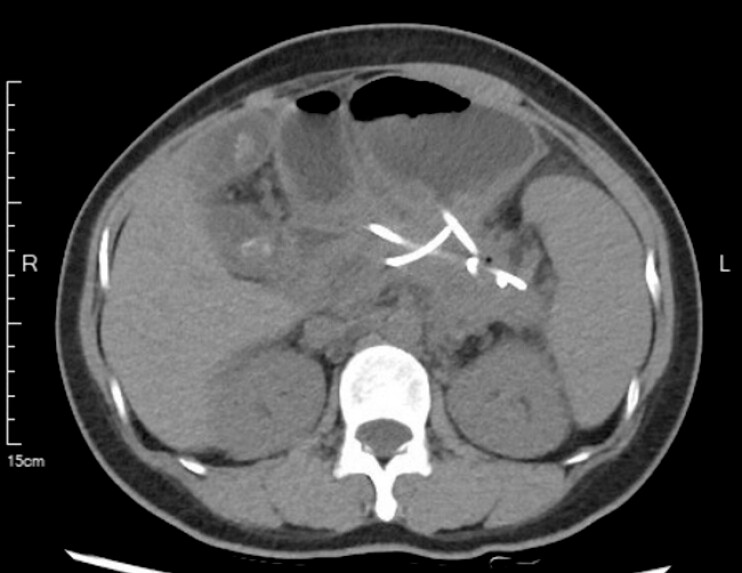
Computed tomography revealed almost disappeared pseudocyst with plastic stents.
